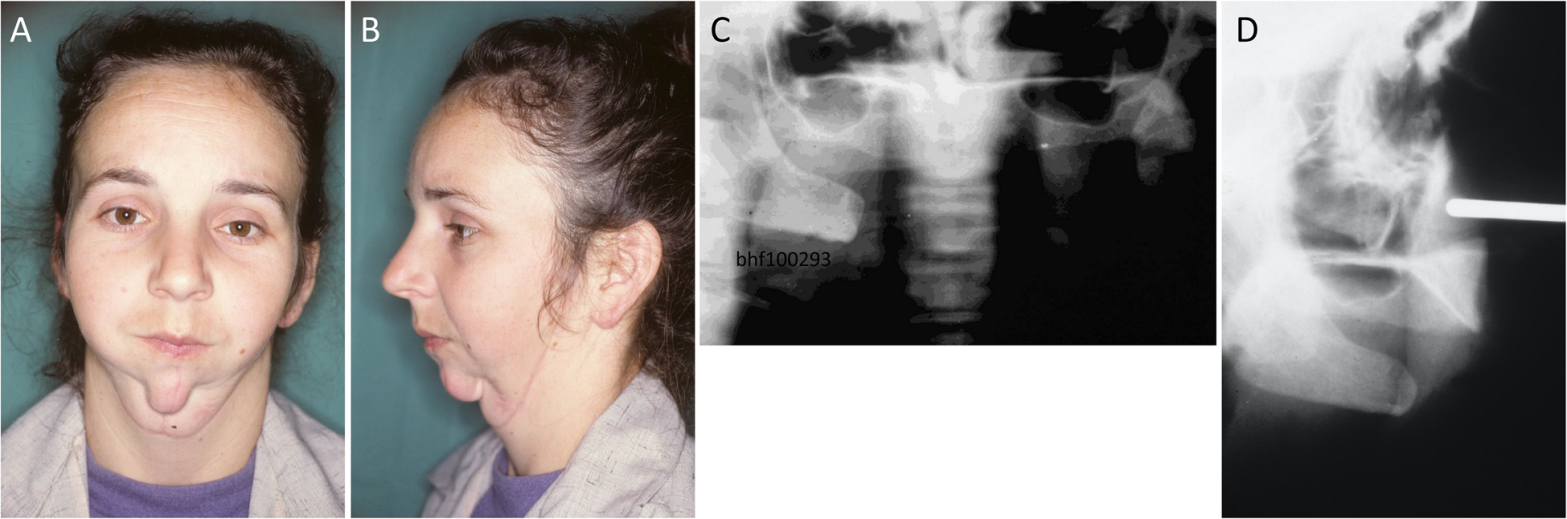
Preoperative images. a, b Frontal and lateral facial aspects of the patient, showing extensive deformity in the lower third of the face as a result of mandibulectomy. c A panoramic radiograph shows, on the right side, the ramus, the angle of the mandible and the retromandibular area; on the left side, remnants of the ascending ramus, from the mandibular foramen on. (Reproduced from Puricelli and Chem, 1985 [11], with permission from RGO – Revista Gaucha de Odontologia, ISSN 0103–6971). d Facial teleradiography
